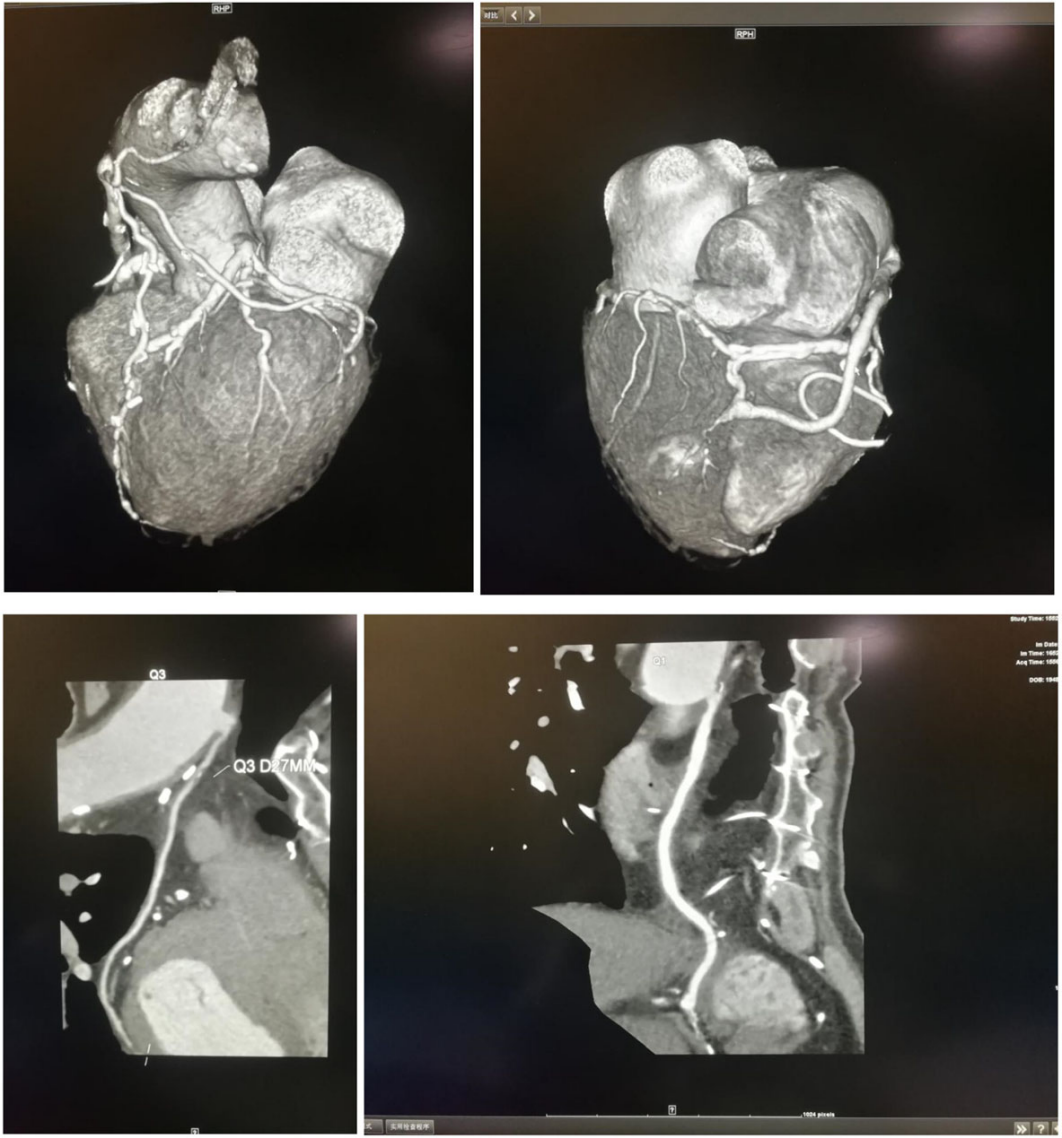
Vascular CTA after CABG in OVH
